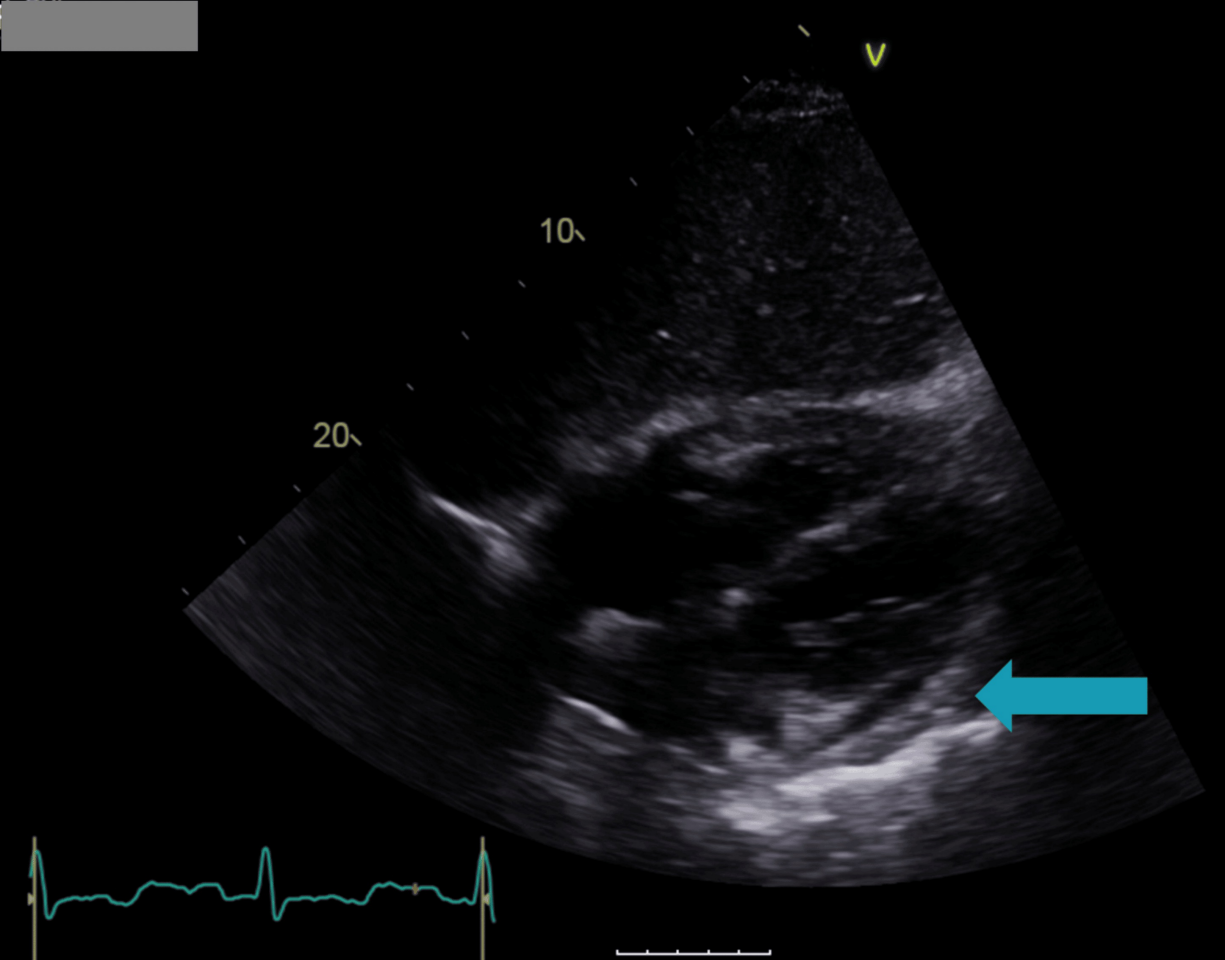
Echocardiographic parasternal long-axis view showing a circumferential anechoic space around the heart, consistent with pericardial effusion.
